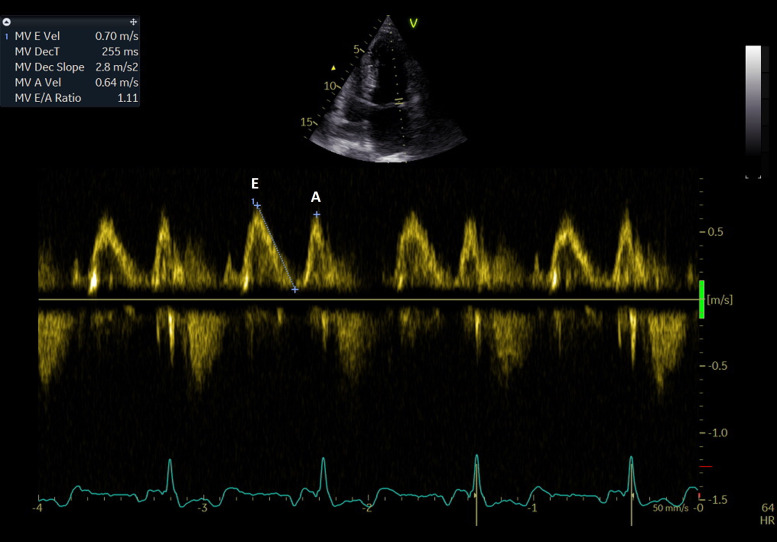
PW Doppler evaluation of the transmitral diastolic flow. E wave
